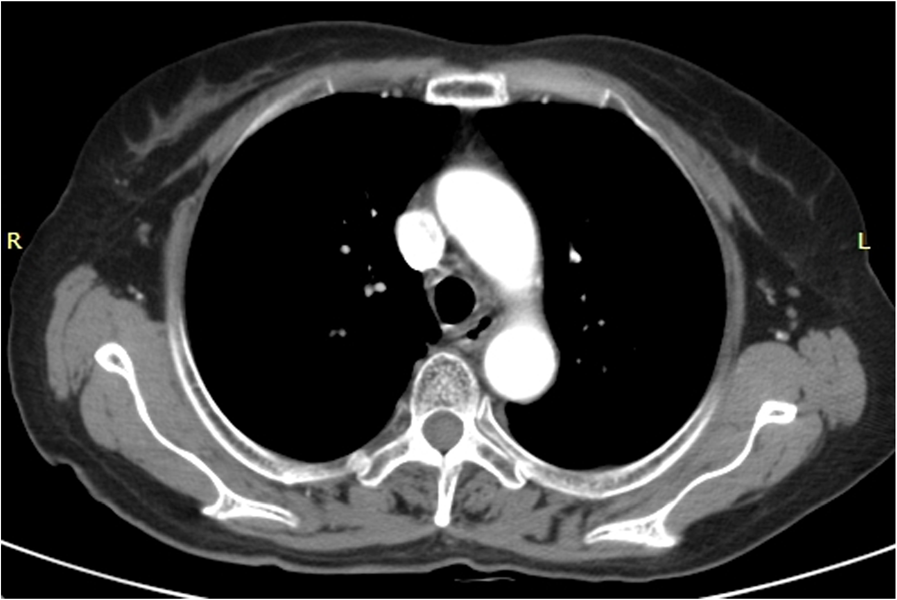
Chest CT scan showing tracheal patency after tracheal stent removal
